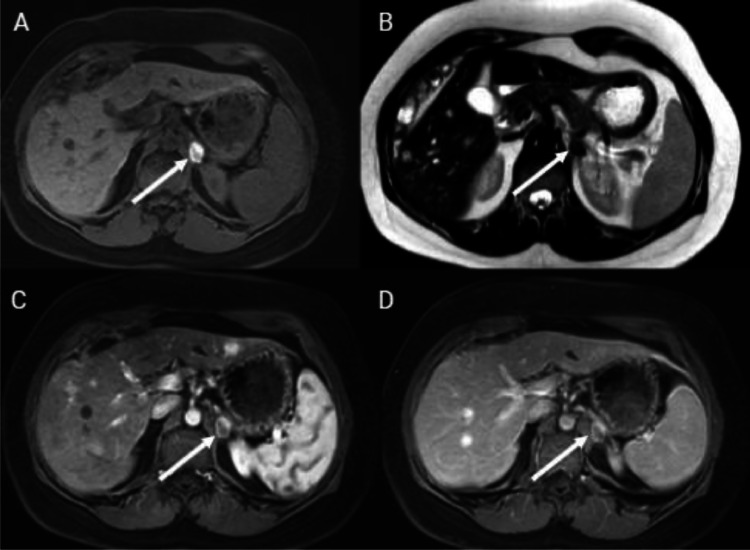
One month post-ablation axial contrast-enhanced magnetic resonance imaging. (A) The T1-fat saturated sequence reveals a high signal lesion consistent with post-ablation coagulative necrosis (arrow). (B) The lesion exhibits low signal intensity in the T2 non-fat saturated sequence (arrow). (C) There is minimal to no enhancement of the lesion in the arterial contrast-enhanced phase, and (D) in the delayed contrast-enhanced phase (arrow).
